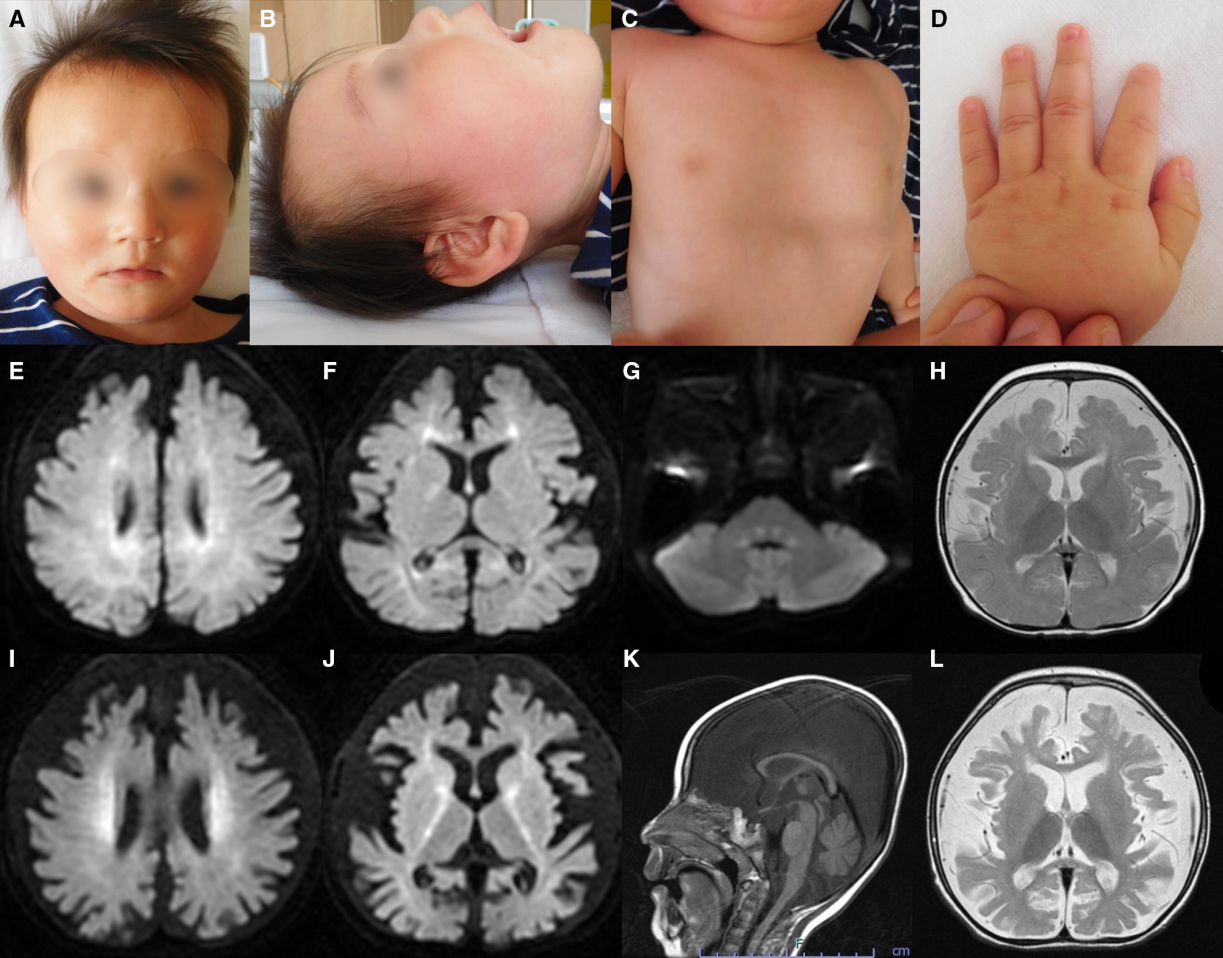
Representative clinical findings. (A–D) Photographs of the patient at ten months of age. Widely spaced eyes, epicanthus, left esotropia, medial sparse eyebrow, pointed chin, and thin upper lip vermilion are observed (A, B). Pectus excavatum (C) and tapered fingers (D) are recognized. (E–L) Brain magnetic resonance imaging (MRI) at ten months of age (E–H) and one year and eight months of age (I–L). Axial diffusion-weighted imaging (DWI) demonstrated elevated signal in the bilateral periventricular white matter, deep and subcortical white matter of the bilateral posterior lobe, posterior limb of the internal capsule, and dorsal part of the pons (E–G). An axial T2-weighted image shows brain atrophy with frontal white matter dominance and diffuse hypointense signal of the thalamus (H) DWI hyperintensities of the bilateral corona radiata and internal capsule are more distinct (I, J). A sagittal T1-weighted image shows atrophy of cerebellar vermis and thinning of the corpus callosum (K). An axial T2-weighted image reveals progressive brain atrophy and no hypointensity in the subcortical white matter. Hypointensity in the anterior limb of the internal capsule is obscured (L).
